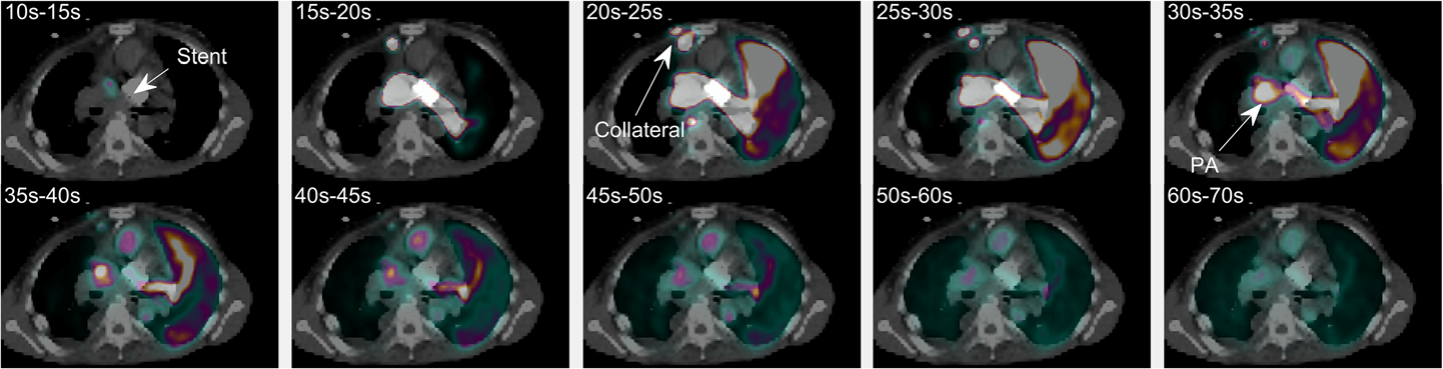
Transaxially fused images of dynamic ^15^O-water PET and CT. A pulmonary artery stent is shown together with collaterals and the pulmonary arteries (PA)
